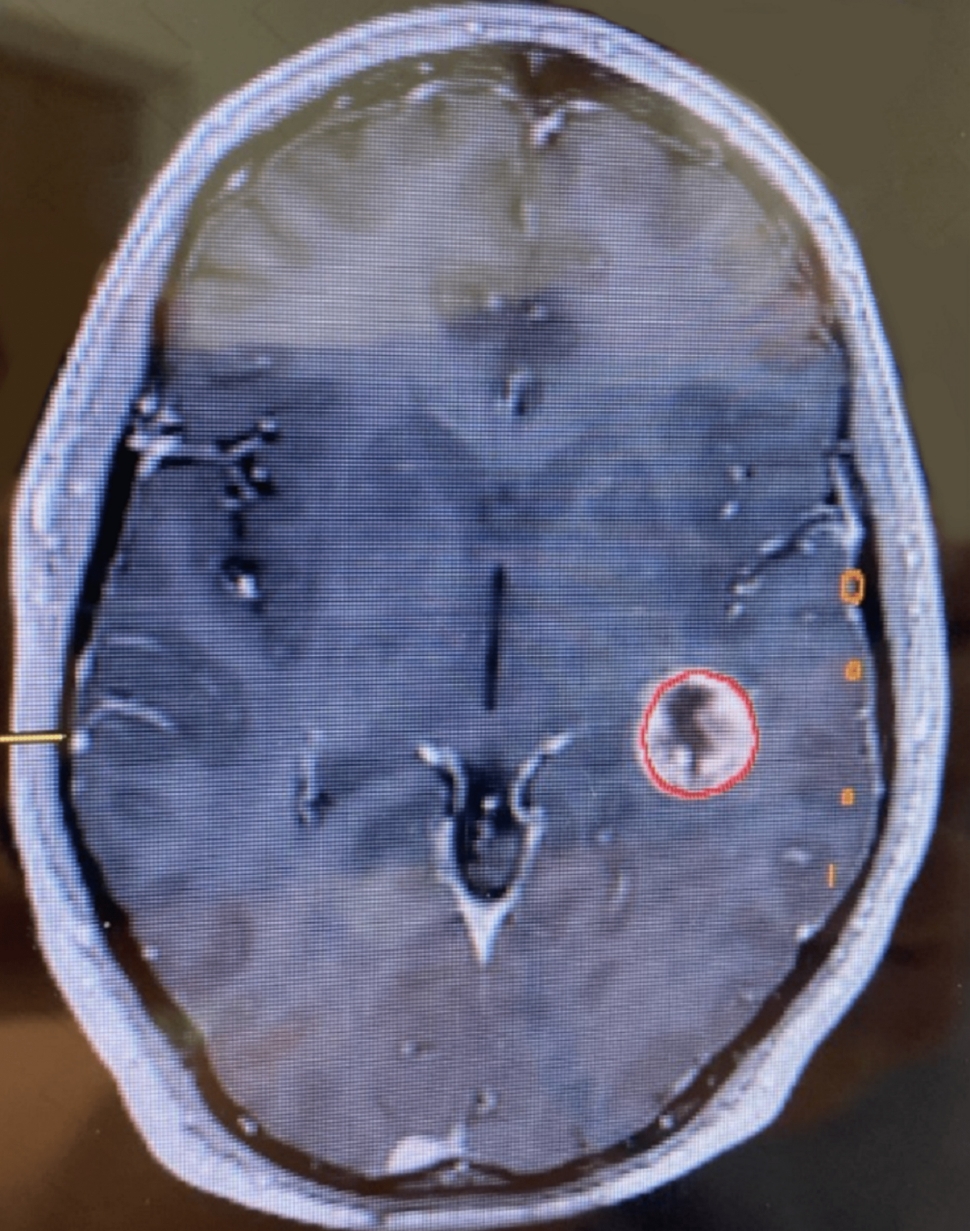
Magnetic resonance image (MRI) showing a tumor in the left cortical region.
